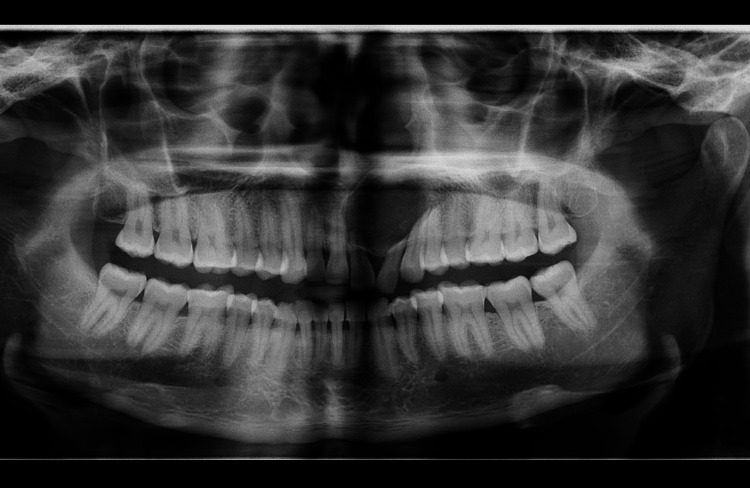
Case 1: OPG showing well-demarcated radiolucency in left maxillary anterior region with Incisor roots deflection and resorption OPG: Orthopantomogram
